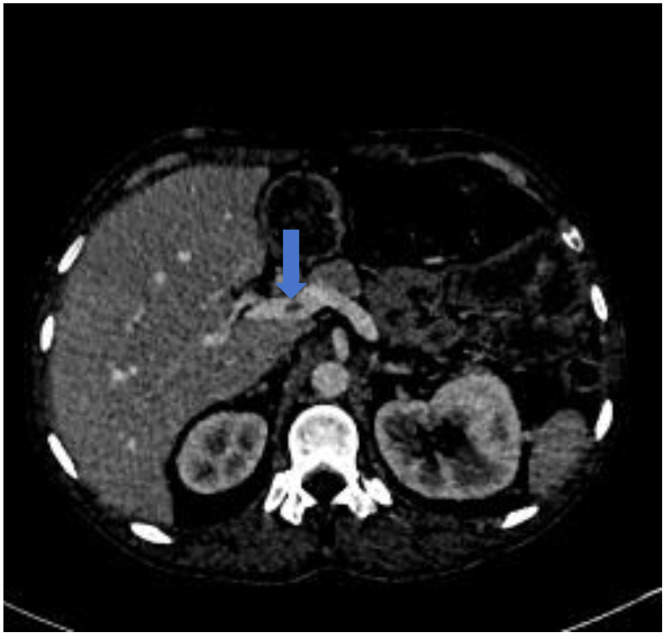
Abdomino-pelvic CT scan: Axial plan showing a thrombus within the porta vein (Blue arrow).
